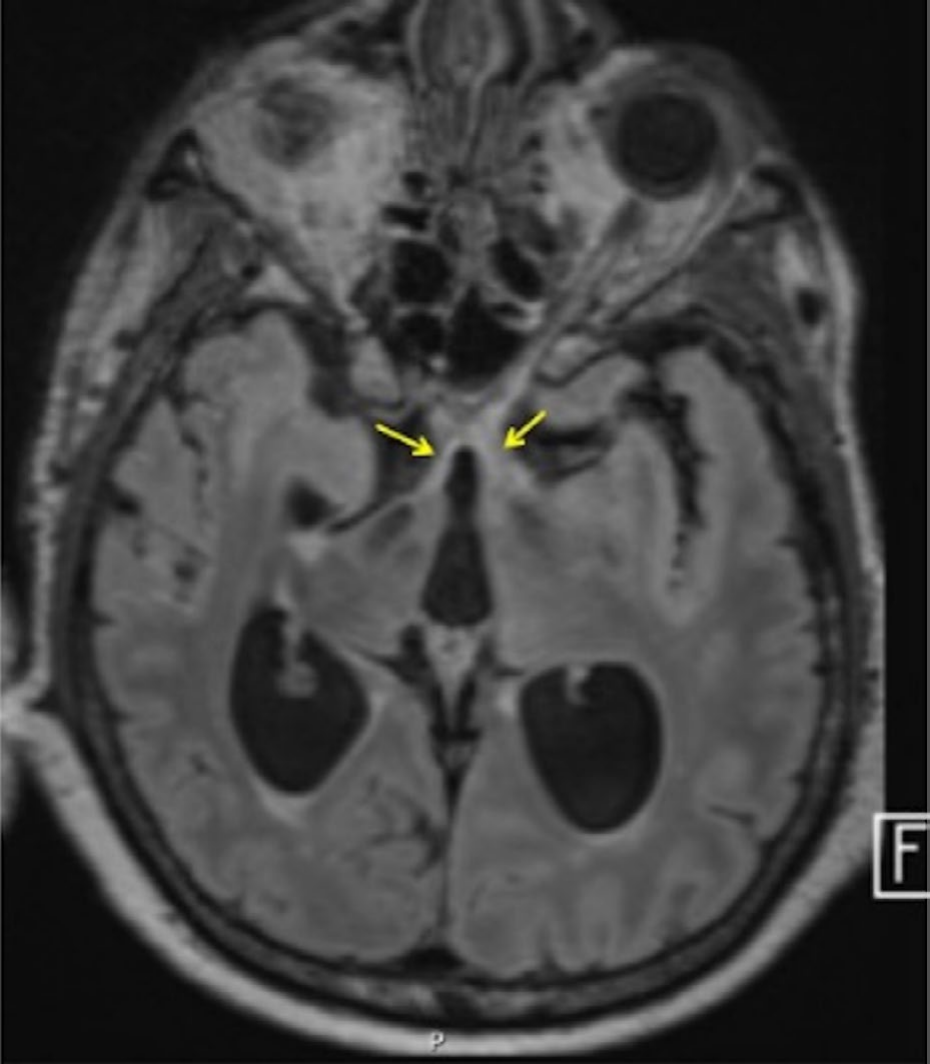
T2/FLAIR: Extension of FLAIR signal abnormality into the post‐chiasmatic optic tracts.
